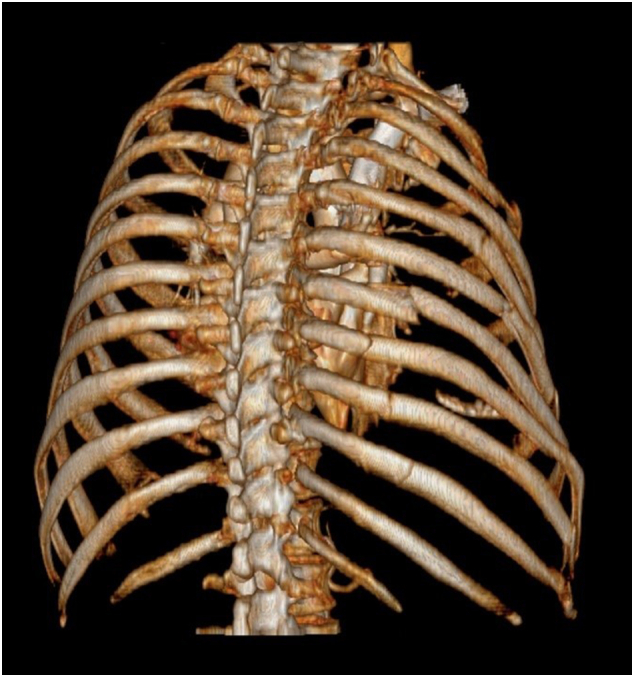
3D Computed tomography rendering demonstrating displaced posterior fractures of ribs five, seven to ten; nondisplaced posterior fracture of sixth rib.
